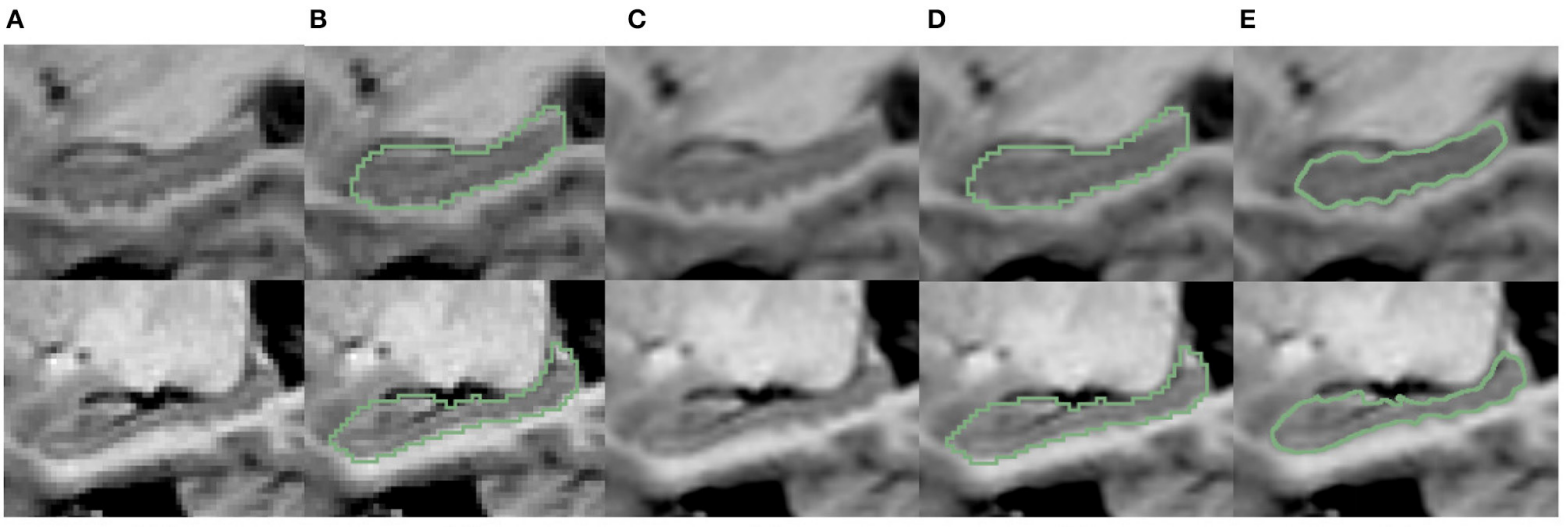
Visualization comparison of hippocampal segmentations at native image resolution vs. fine scale in IXI dataset. (A) The MR image of the hippocampus at native resolution. (B) The segmentation of hippocampus at native resolution; (C) shows the MR images after interpolation; (D) shows the native resolution hippocampus segmentation overlaid on the fine-scale MR image after interpolation; (E) shows the fine-scale hippocampus segmentation overlaid on the fine-scale MR image.
